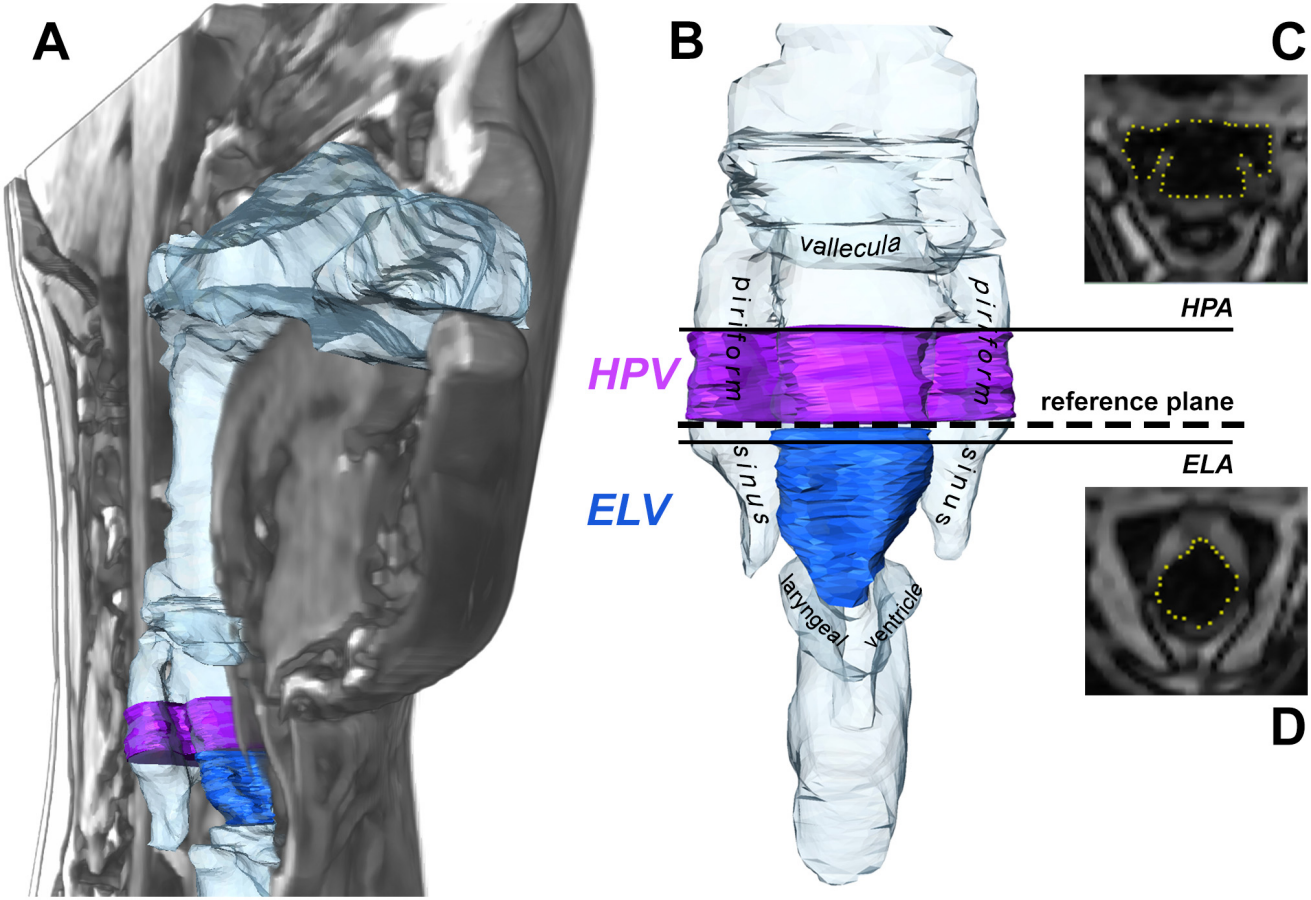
Visualization of the analyzed vocal tract measures. (A) 3D model of the complete vocal tract in a right-oblique projection within a midsagital section of the corresponding MRI data. (B) 3D model of the lower vocal tract showing the location of the reference plane at the top of the arytenoids, the selected volume measures: in purple: hypopharyngeal volume (*HPV* = volume from reference plane 10 mm upwards), in blue: endolaryngeal volume (*ELV* = volume from reference plane to lower ventricular folds) as well as the position of the area measures: hypopharyngeal area (*HPA*) and endolaryngeal area (*ELA*) with the corresponding segmented MR images (*HPA*/(C) and *ELA*/(D)).
